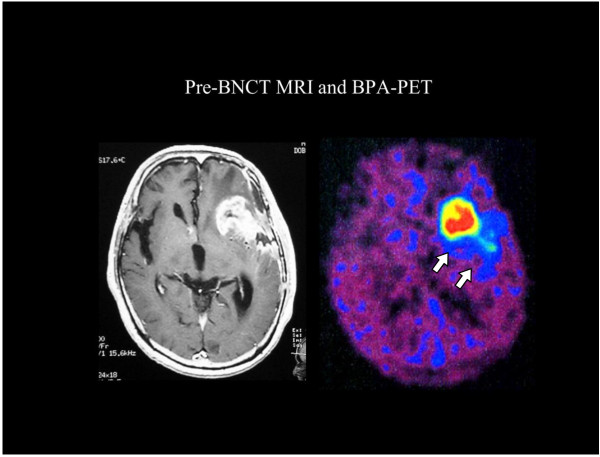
Contrast-enhanced T1-weighted MRI of representative glioblastoma patient and^18^ F-labeled BPA-PET image after initial debulking surgery. The patients received ^18^ F-BPA-PET to assess the distribution of BPA and to estimate the boron concentration in tumors before BNCT without direct determination of boron concentration in the tumor. The lesion to normal brain (L/N) ratio of the enhanced tumor was 7.8 in this case. Note that even the periphery of the main mass, i.e., the infiltrative portion of the tumor, showed BPA uptake. The L/N ratio of BPA uptake can be estimated from this study and dose planning was done according to this L/N ratio, and if the L/N ratio was more than 2.5, then BNCT was initiated. ^18^ F-BPA-PET accurate BPA provided an accurate estimate of the accumulation and distribution of BPA as previously reported [64,65].
